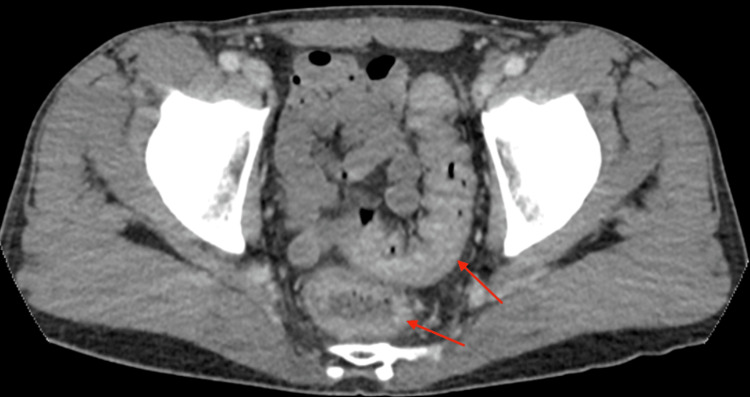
Axial CT imaging showing coloproctitis Axial reformat image of the abdomen and pelvis with IV contrast demonstrates mild wall thickening and enhancement involving the rectum and sigmoid colon, consistent with colitis.
